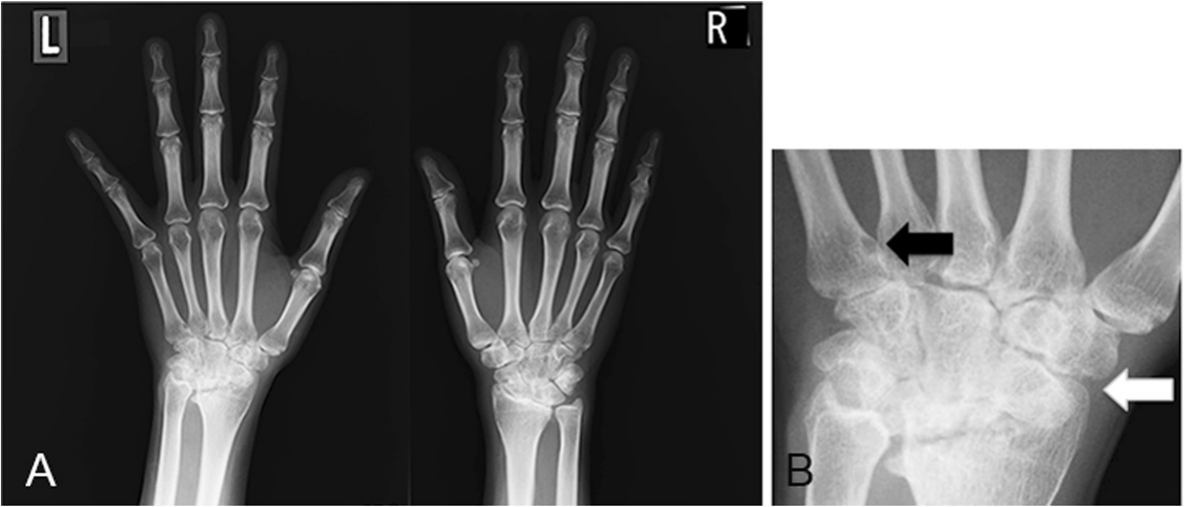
X-ray of bilateral fingers and wrists at the diagnosis: A)
